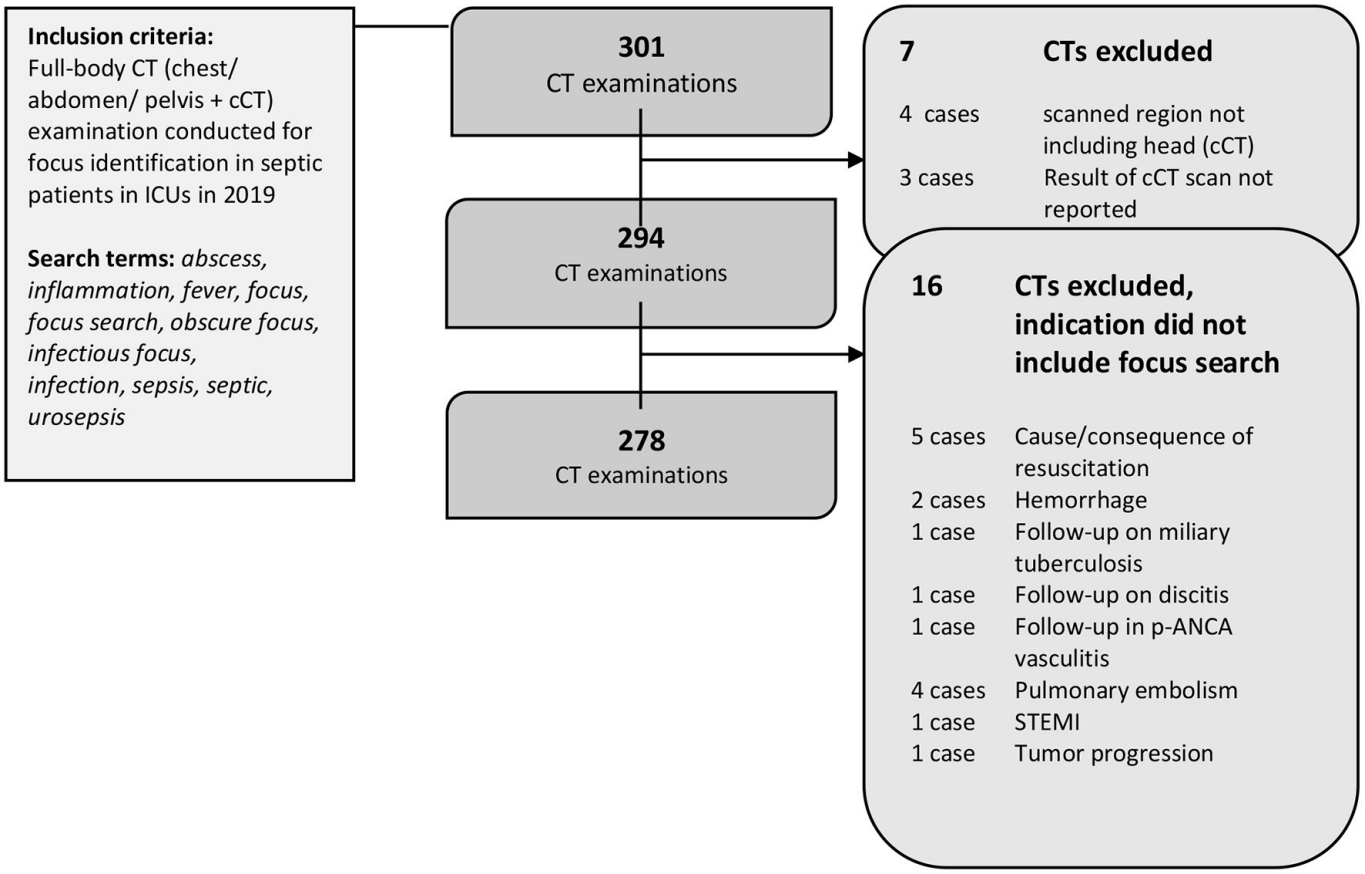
Patient flow. 301 CT reports were identified by the full-text search. Seven CTs were excluded as the required organ regions were not fully examined. Another 16 CTs were excluded after the analysis due to indications other than focus search. A final 278 CT reports were included in the analysis.
